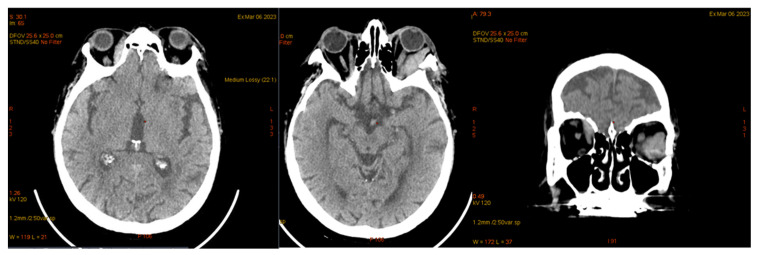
Cerebral computed tomography—same characteristics as the previous one.
